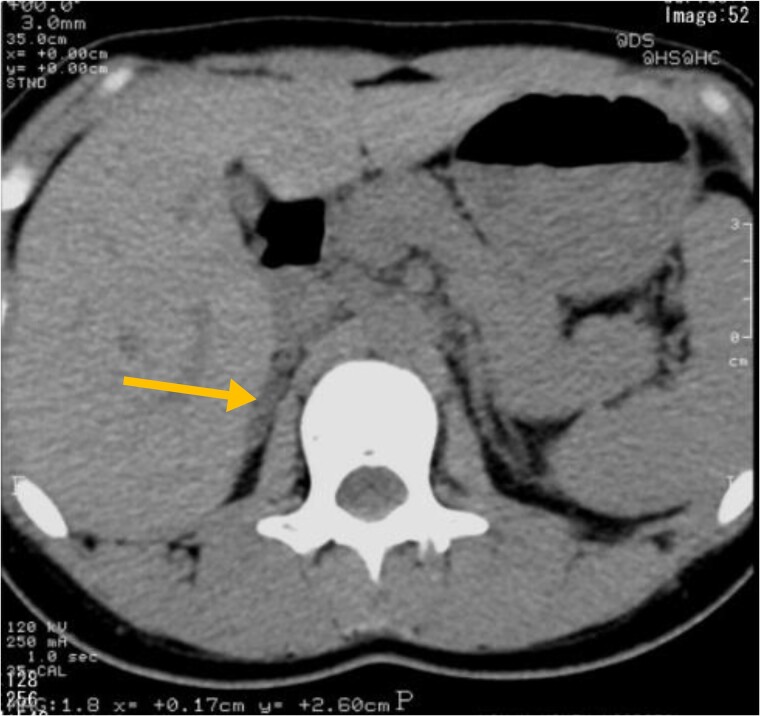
CT image of the abdomen. The arrow indicates the right adrenal gland. No abnormalities were identified on CT. Abbreviation: CT, computed tomography.
